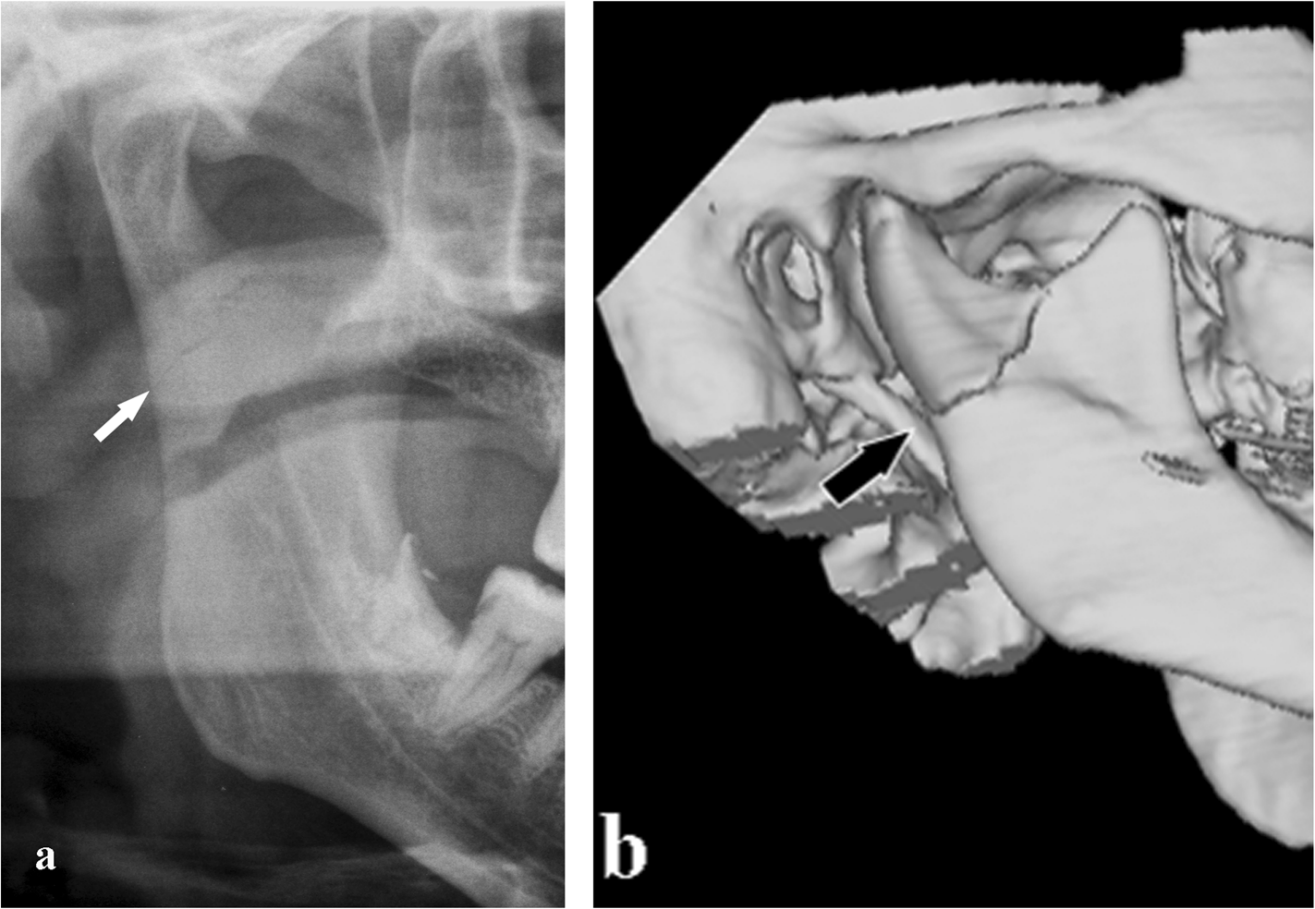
Condylar process fracture. No deviation. a Cropped panoramic radiograph. b 3D computed tomography reformation of the same patient. The bone fragments are in line and close to each other (arrows)
